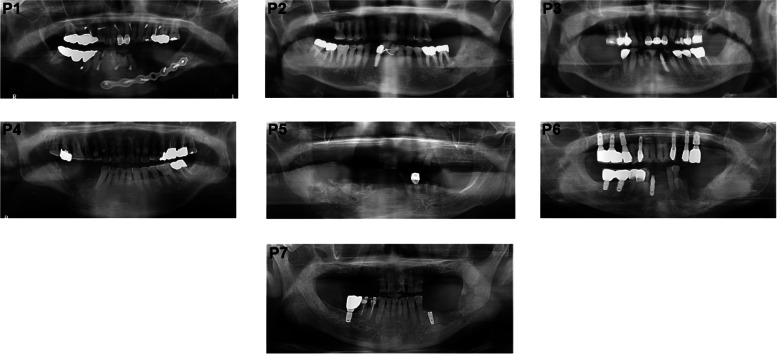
Preoperative orthopantomogram X-rays of the patients
